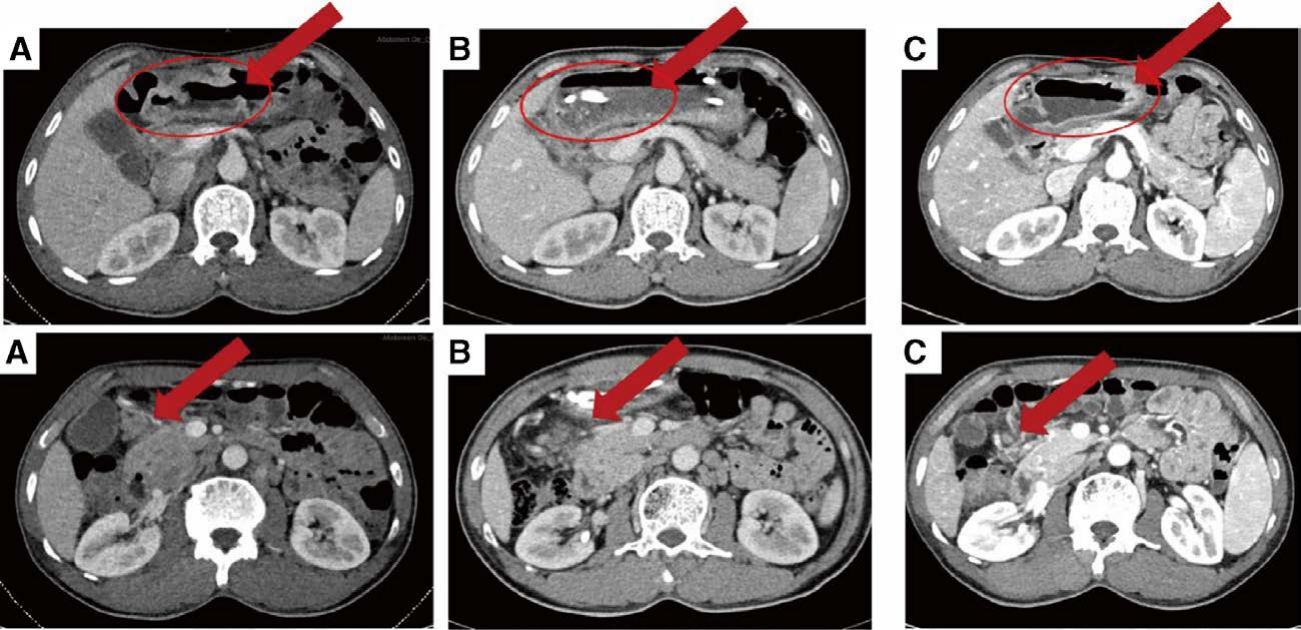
(A) Enhanced CT of abdomen at admission showed irregular thickening of the gastric wall and a 2.2cm*2.0cm enlarged lymph. (B)&(C) Enhanced CT with dynamically review during neoadjuvant chemotherapy revealed the regression of the lesion.
